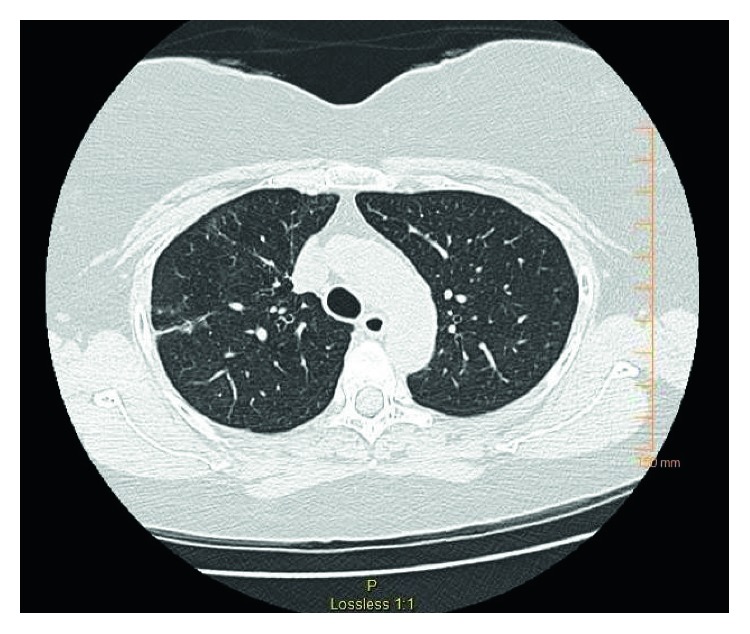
CT chest showing resolution of earlier chest lesion.
